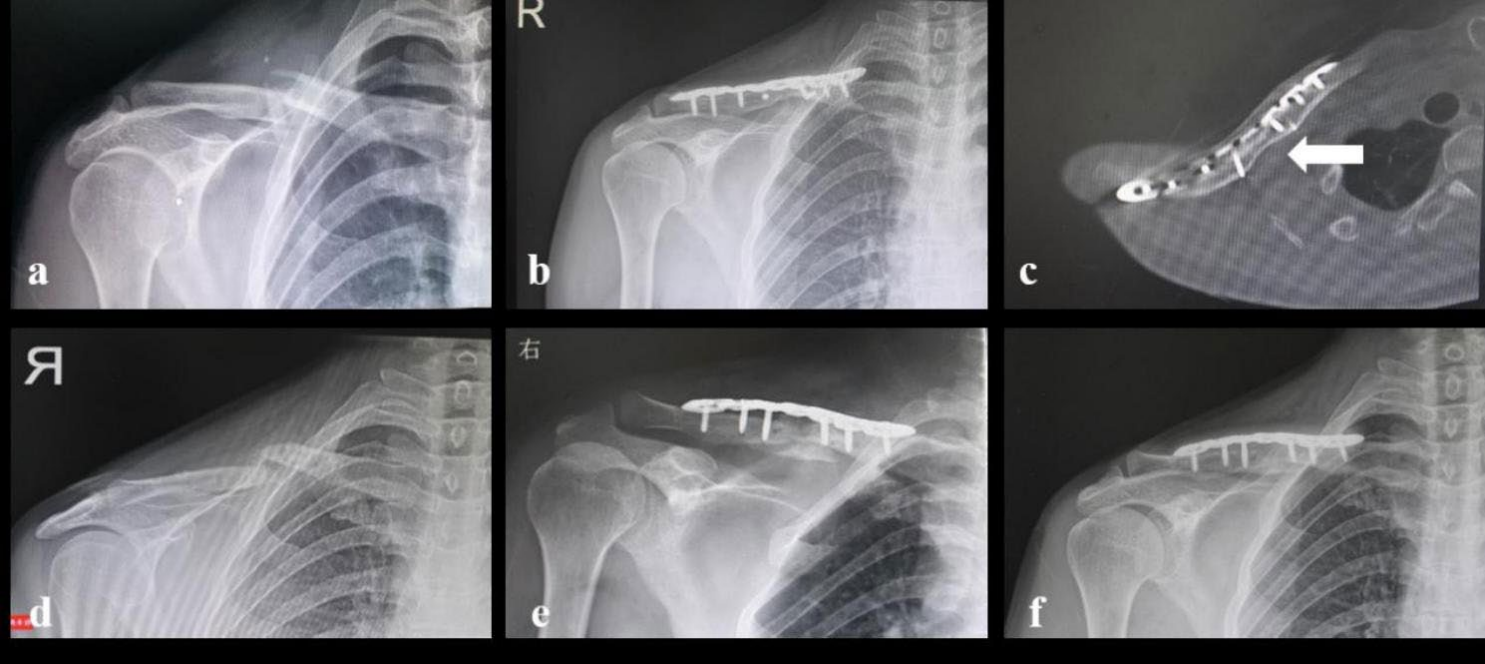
A female patient (54 years old) had a midshaft clavicle fracture (Robinson 2B2) after a fall to ground (a). She received ORIF and achieved bone union (b) and insisted on implant removal though CT scan showed that the bone quality at previous fracture site was not strong as that of surroundings (c, white arrow, 108 weeks after primary operation). She had implant removed and unfortunately refractures occurred at previous fracture site 30 days after implant removal (d). Reoperation with autogenous iliac bone graft (black arrow) was applied (e) and she achieved bone union 3 months later (f)
